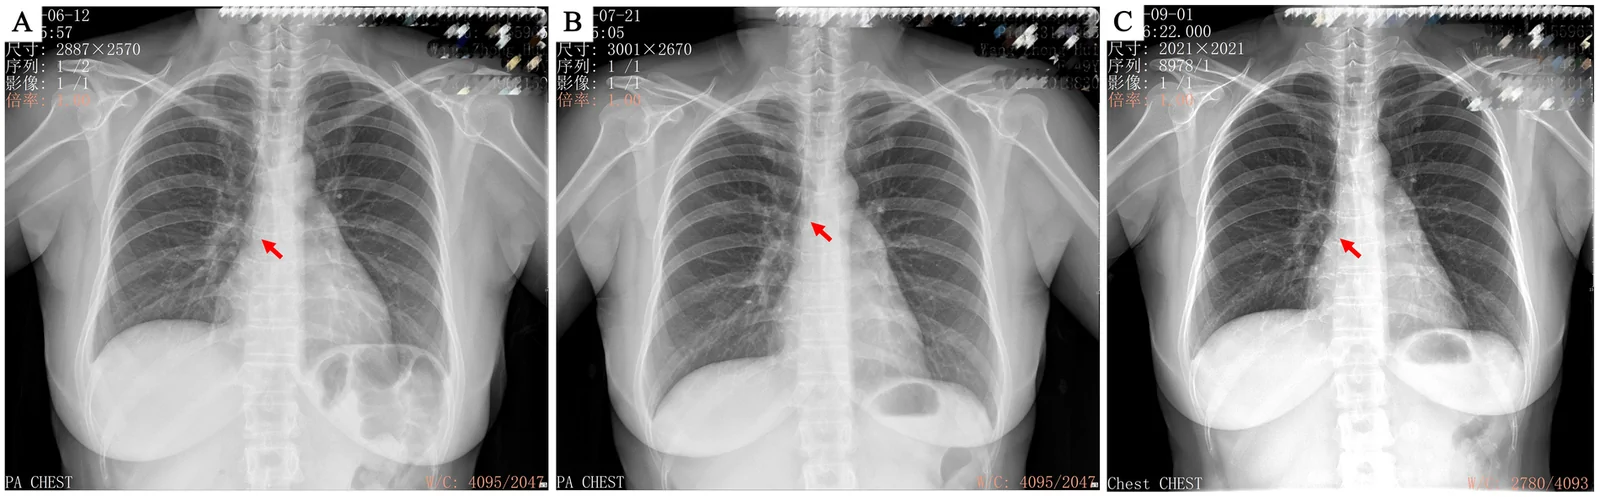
Chest radiographs obtained during TIVAP use, showing sequential changes in catheter tip position: (A) immediately after TIVAP implantation, with the catheter tip at the superior border of T8; (B) during the third cycle of chemotherapy, with the tip at the T6–T7 level; (C) during the fifth cycle of chemotherapy, with the tip at the T8 level.
